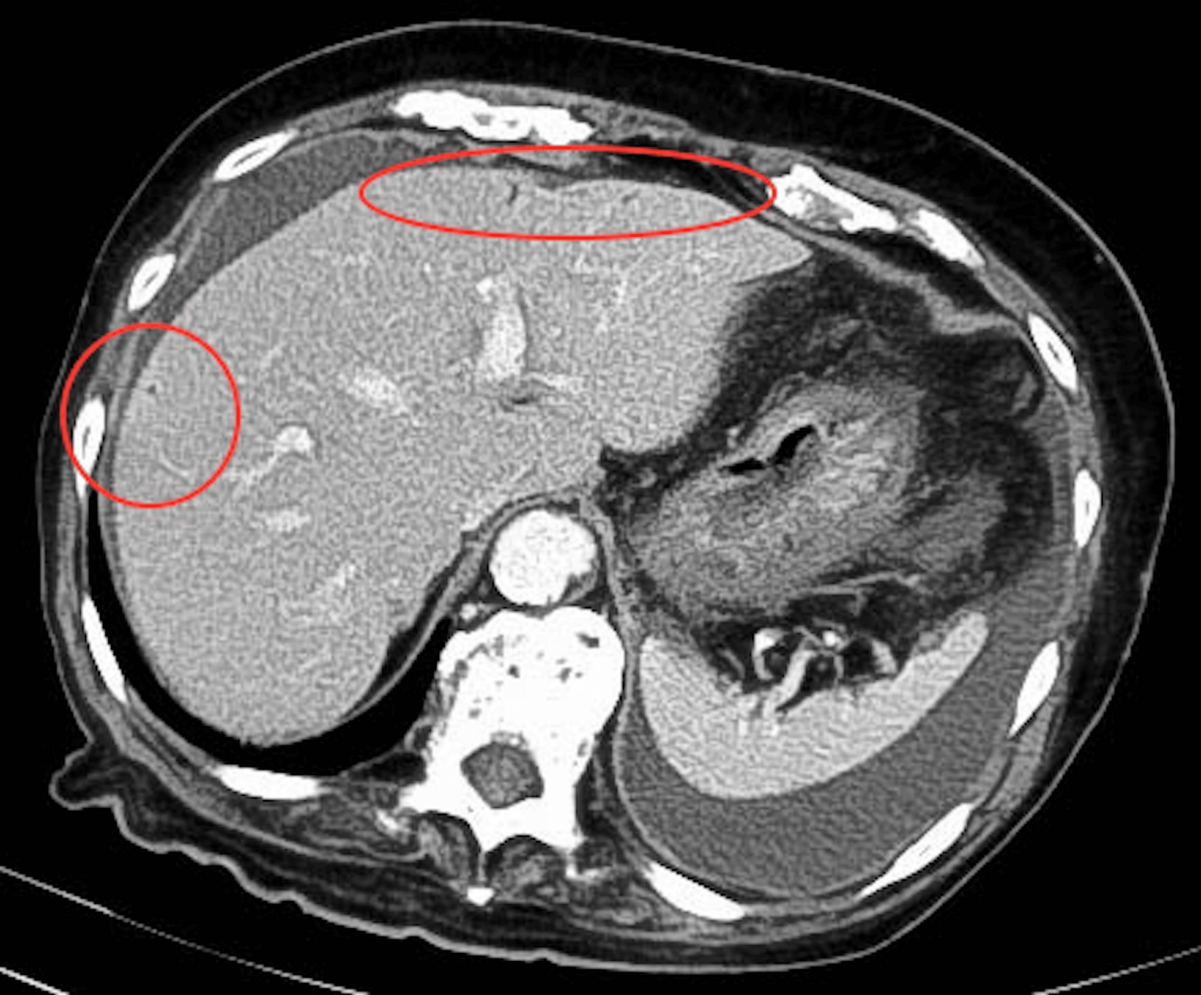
Computed tomography findings of portal vein gas Contrast-enhanced computed tomography of the upper abdomen demonstrates only a few small gas foci in the portal vein (red circle), less evident than on ultrasound, in the same patient.
